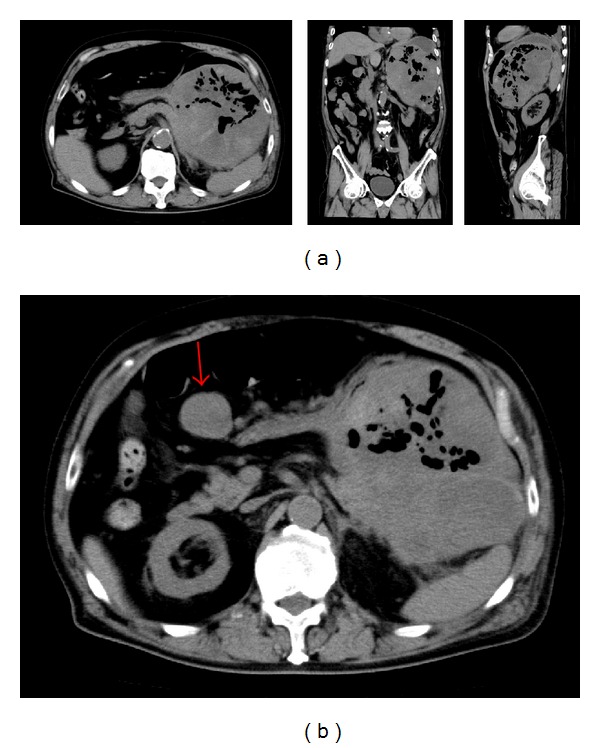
(a) Abdominal computed tomography (CT) scan showing a giant mass with central necrosis in the stomach. (b) Abdominal computed tomography scan showed a peritoneally disseminated lesion, measuring 30 × 30 mm in size (arrow).
